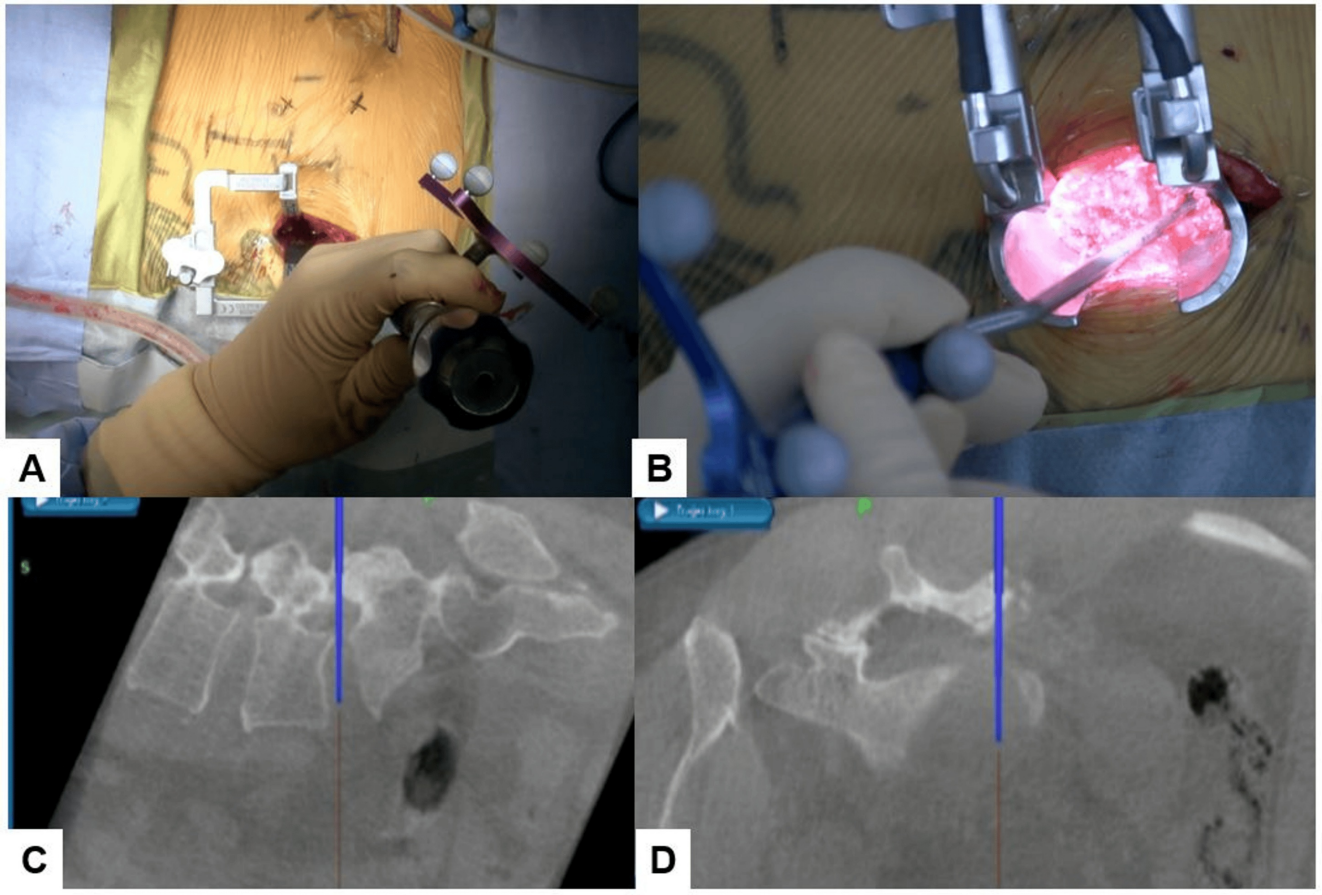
Surgical technique of C-arm-free TLIF Place the reference frame percutaneously into the ilium near the posterior superior iliac spine and capture images with the O-arm. Use the navigation to determine the pedicle screw entry point and create a mini-open incision at the midpoint between the facet joint and the pedicle screw entry point on the side intended for cage insertion. On the cage insertion side, perform tapping up to the screw hole (A); on the contralateral side, insert the pedicle screw. Under navigation, resect the facet joint and intervertebral disc (B), then insert and rotate a banana-shaped cage without the use of a C-arm. After cage placement, use a navigated pointer to approximate the position verification (C). Confirm final positioning with X-ray imaging (D). TLIF: transforaminal lumbar interbody fusion
